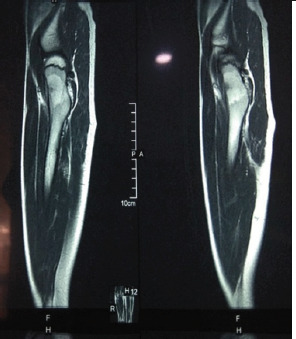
MRI showed subcortical intramedullary lesion with perilesional edema.
